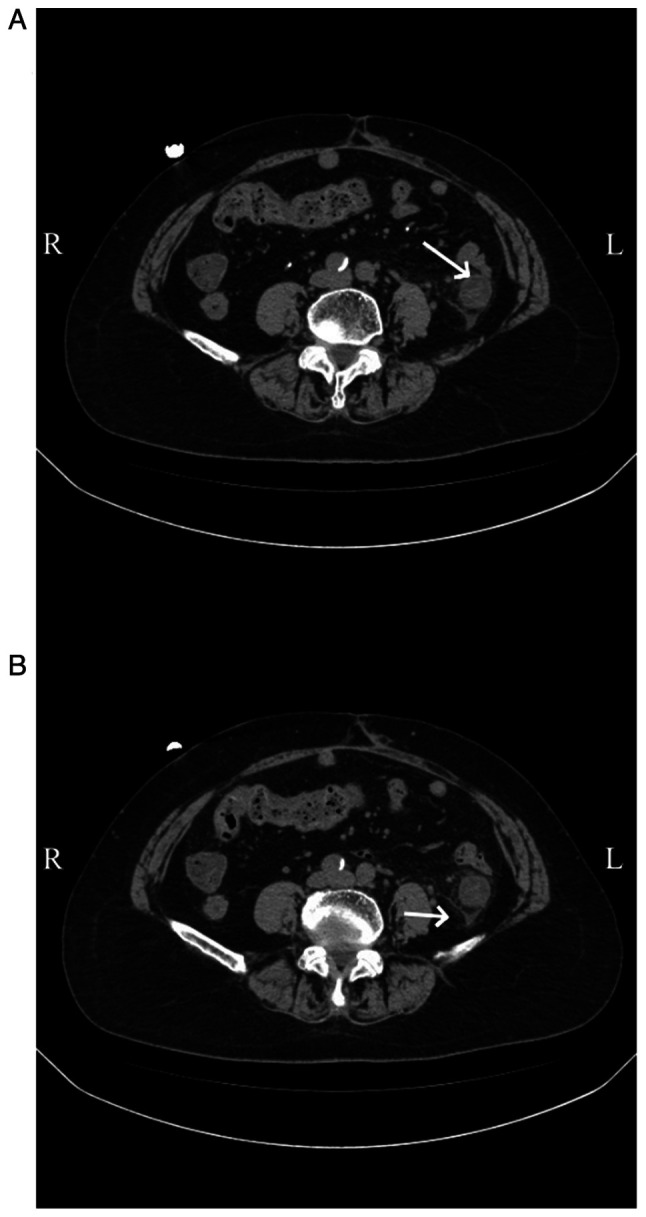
Abdominal computed tomography scan images of a patient with exudation around the descending colon accompanied by an abdominopelvic effusion. (A) Edema (arrow) in the descending colon with surrounding exudation. (B) Abdominopelvic effusion (arrow). R, right side of the patient; L, left side of the patient.
